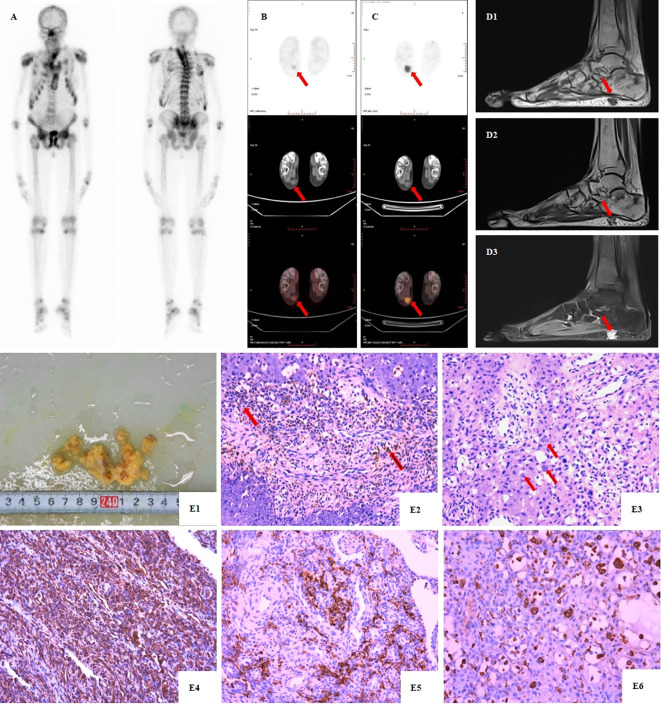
(A) Whole body bone scan showed increased multi bone metabolism with changes in bone density, and multiple old fractures.; (B) PET-CT showed a subcutaneous nodule in the right sole, with mild increase in FDG metabolism (SUVmax 1.74); (C)
